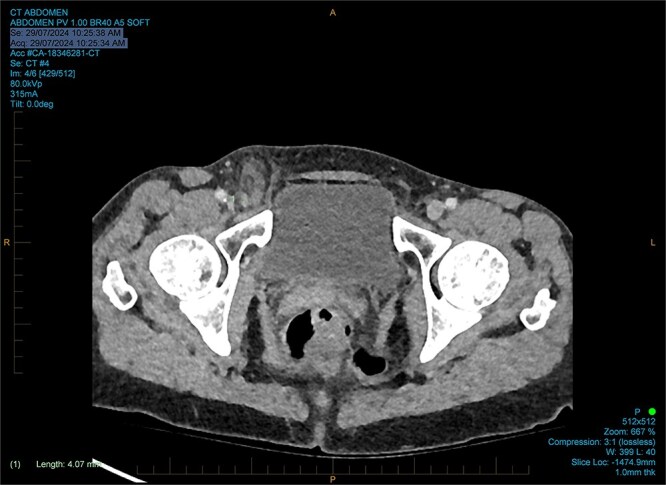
Coronal CT reported as an Amyand’s hernia.
